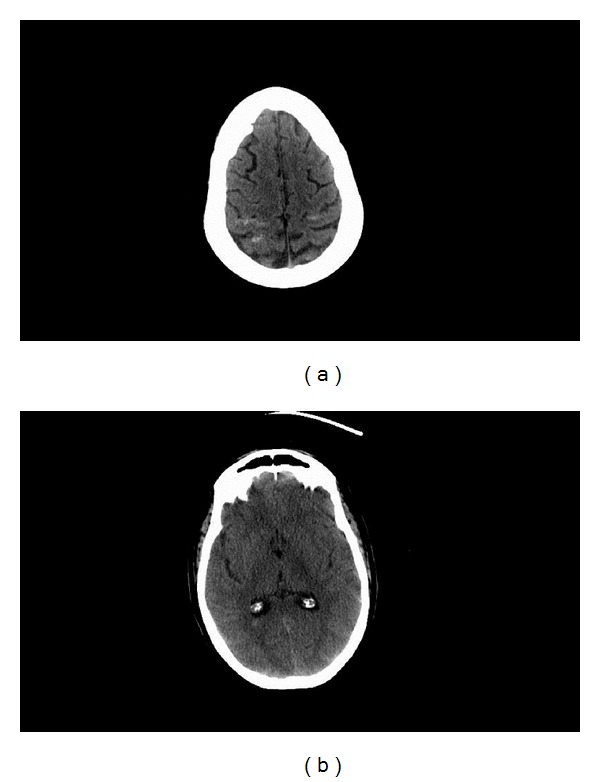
Axial CT head images without contrast on postoperative day 0 demonstrating subarachnoid hemorrhage bilaterally in the posterior frontoparietal regions (a) and hemorrhage in the occipital horn of the right lateral ventricle (b).
